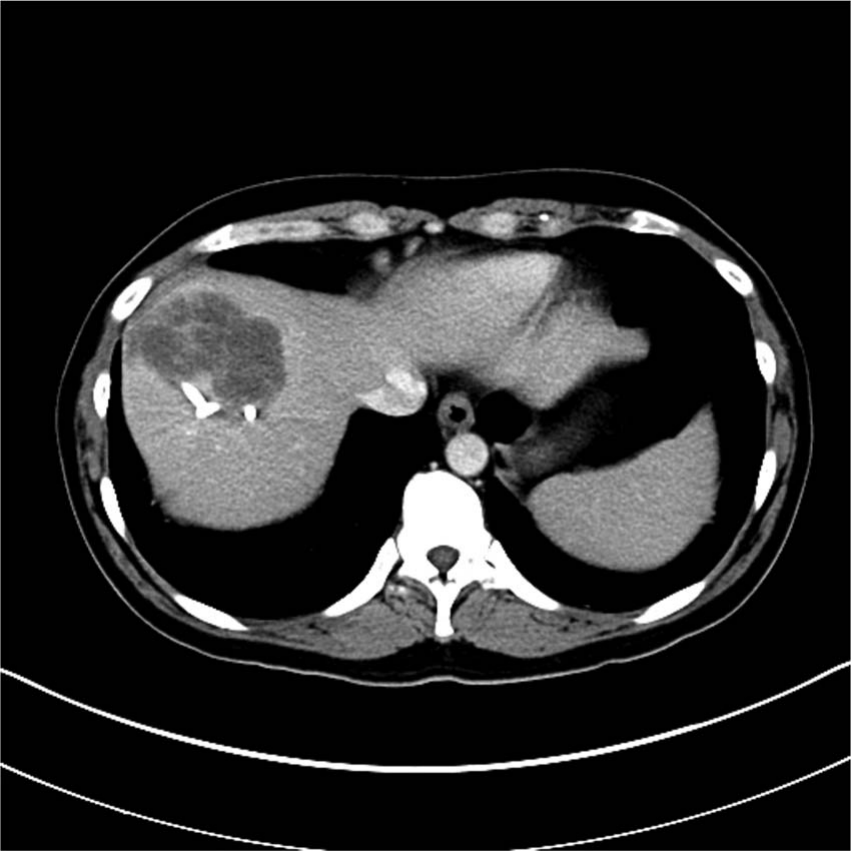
Axial view of CT image of abdomen.
